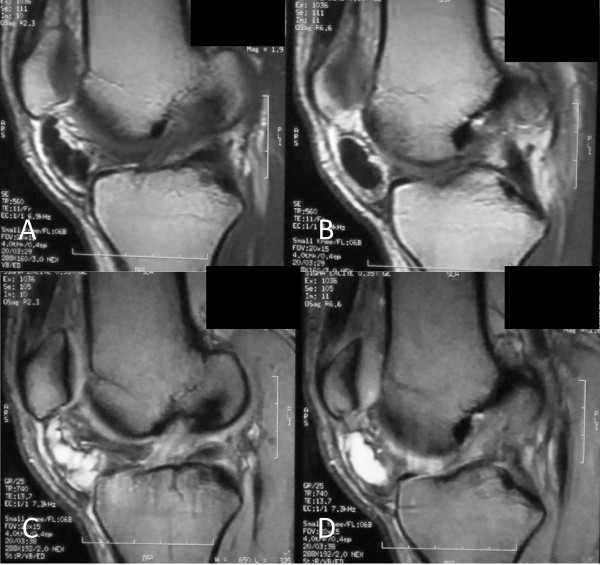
Sagittal magnetic resonance images of the knee. (A, B) Sequential T1-weighted images of a cystic lesion with low signal intensity inferior to patella within the Hoffa's fat pad. (C, D) Sequential T2-weighted images, at the same level, of a well-demarcated, multilobular cystic mass with high signal intensity. Note the extrasynovial intra-articular location of the lesion.
